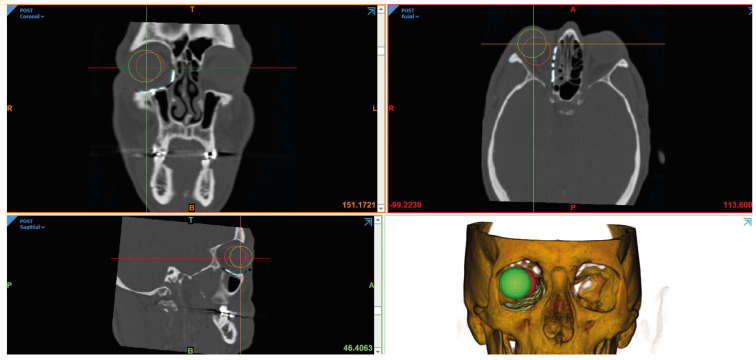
Defining globe position on pre- and post-operative CT. Globe position was defined by fitting a sphere to the globe’s circumference using Mimics Medical software (Materialise^®^, Leuven, Belgium). The pre-operative sphere is represented in red, while the post-operative sphere is shown in green. In this patient, an anterior–lateral repositioning following PSI reconstruction was observed.
